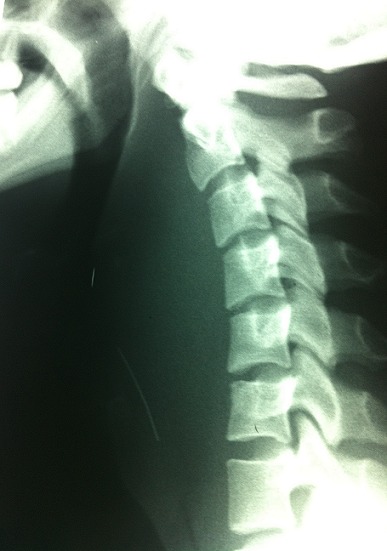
Radiography of the cervical spine showing an aspect of retropharyngeal collection and the presence of a radio opaque foreign body
